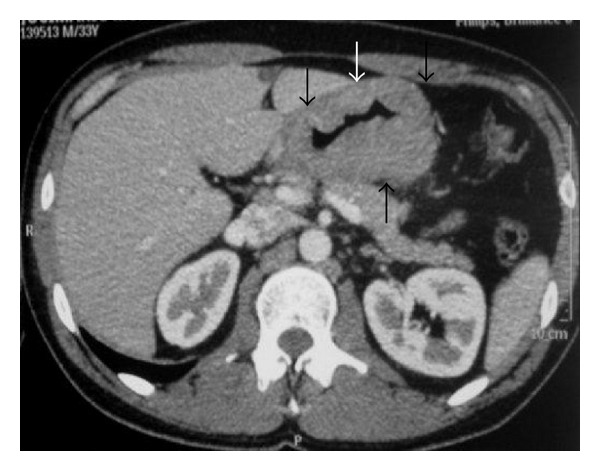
A 33-year-old man with gastric lymphoma. Axial contrast-enhanced abdominal CT demonstrates irregular thickening of the wall of the stomach (arrows).
